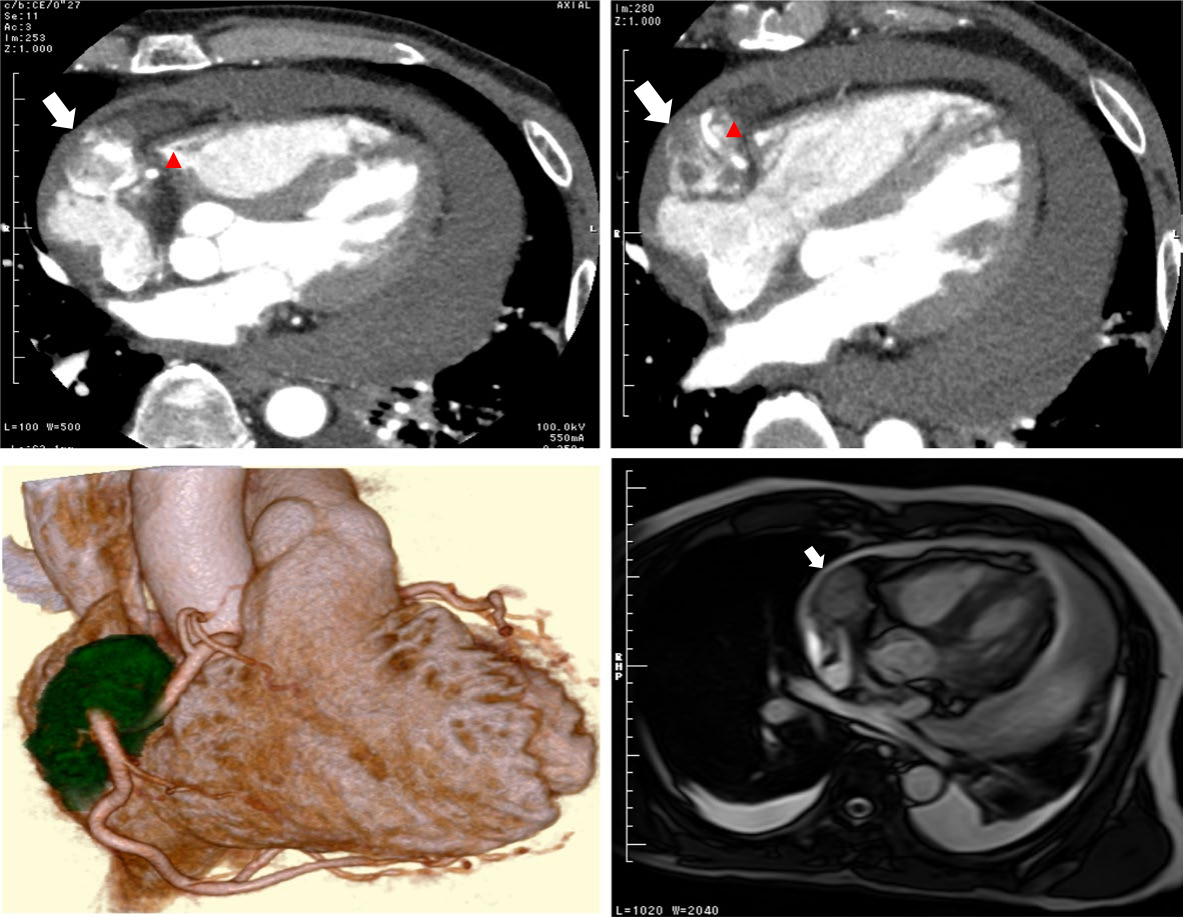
Contrast-enhanced CT images revealing a massive pericardial effusion and a 40-mm enhanced mass (white arrow) adherent to the right atrial wall and involving the RCA (red arrow head). MRI showing the same mass (white arrow), which exhibited an isointense to high signal intensity on T2-weighted images
